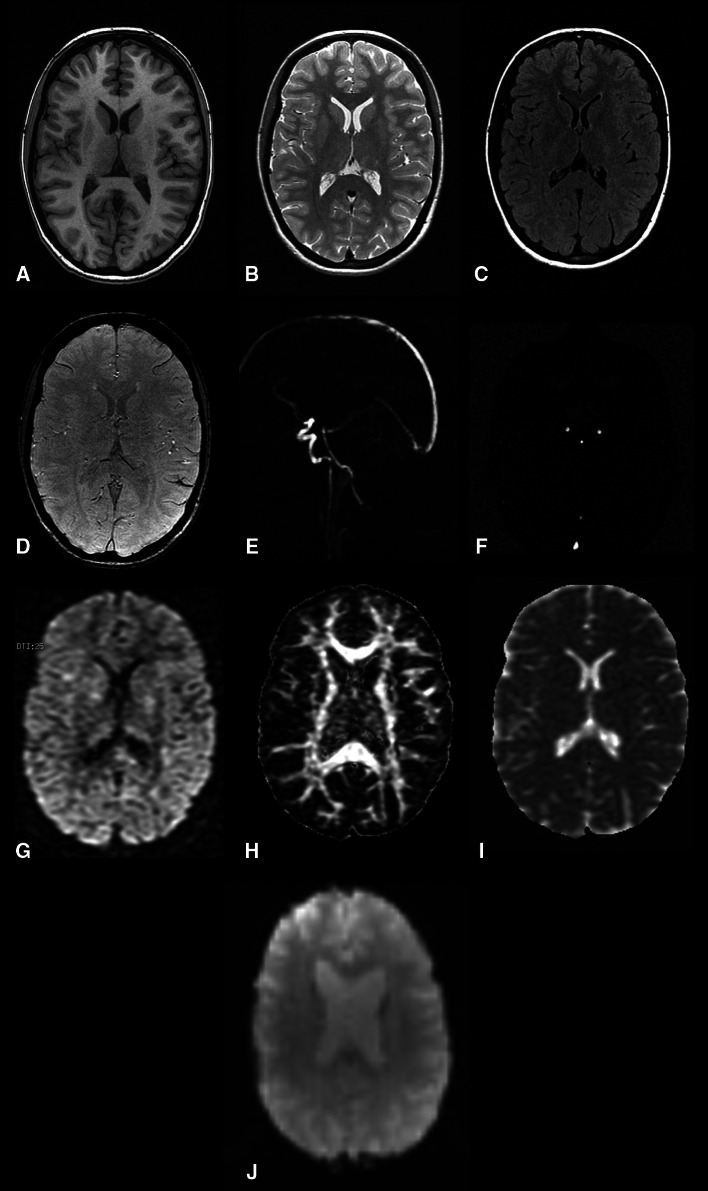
Depiction of the images acquired using the MRI protocol. First row: T1-weighted (*a*), proton-density-weighted (*b*), and fluid attenuated inversion recovery (*c*) images. Second row: T2*-weighted (*d*) image, sagittal scout for the 2D phase contrast measurement (*e*), and the resulting flow image (*f*). Third row: example of an image acquired using diffusion weighted imaging (*g*), map of fractional anisotropy (*h*), map of mean diffusivity (*i*), and resting-state functional MRI (*j*)
